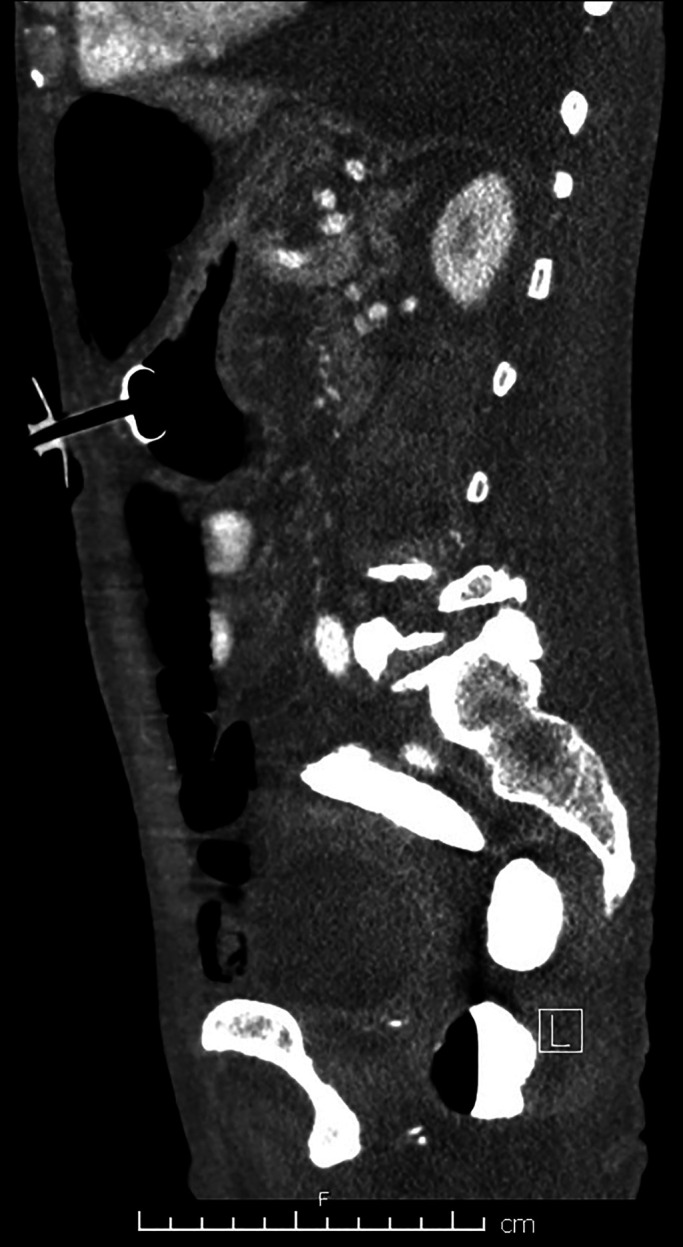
Postprocedure abdominal computed tomography (sagittal projection) confirming successful placement of the percutaneous endoscopic gastrostomy tube.
